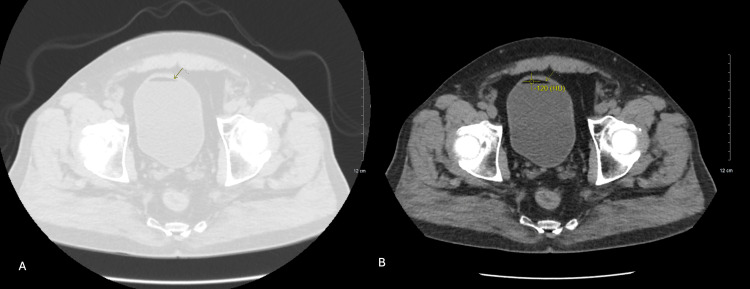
Follow-up CT, axial view, in the lung window (A) and with HFU measurement (B) The lung window (image A) authenticates that the radiolucency in the bladder is not air, and the Hounsfield unit (HFU) measurement of -120 (image B) verifies the lipid-like fluid, confirming chyluria.
